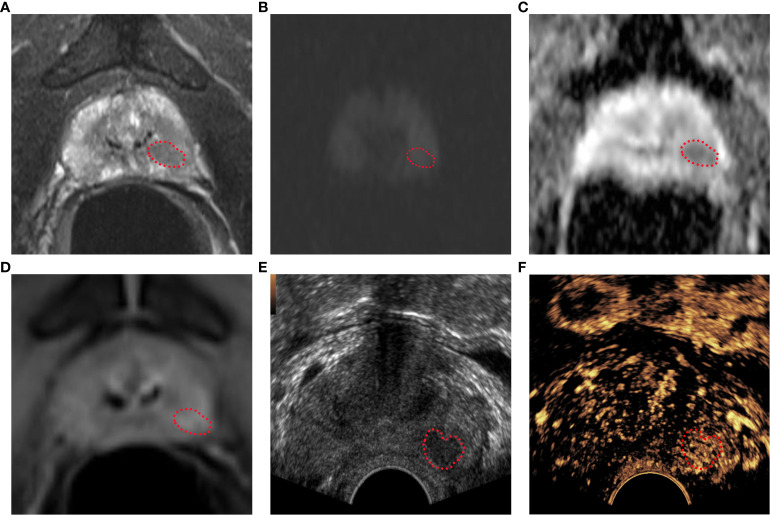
Bp-MRI score, PI-RADS v2.1 score, and CEUS-BpMRI score results display. Bp-MRI score was 3. PI-RADS v2.1 score was 4. The CEUS-BpMRI score was 4. The lesion was marked with a red dashed line. (A) T2WI score was 4. (B) DWI (B value 1000) and (C)ADC. (D) DCE: positive. (E) Conventional ultrasound. (F) CEUS: positive.
